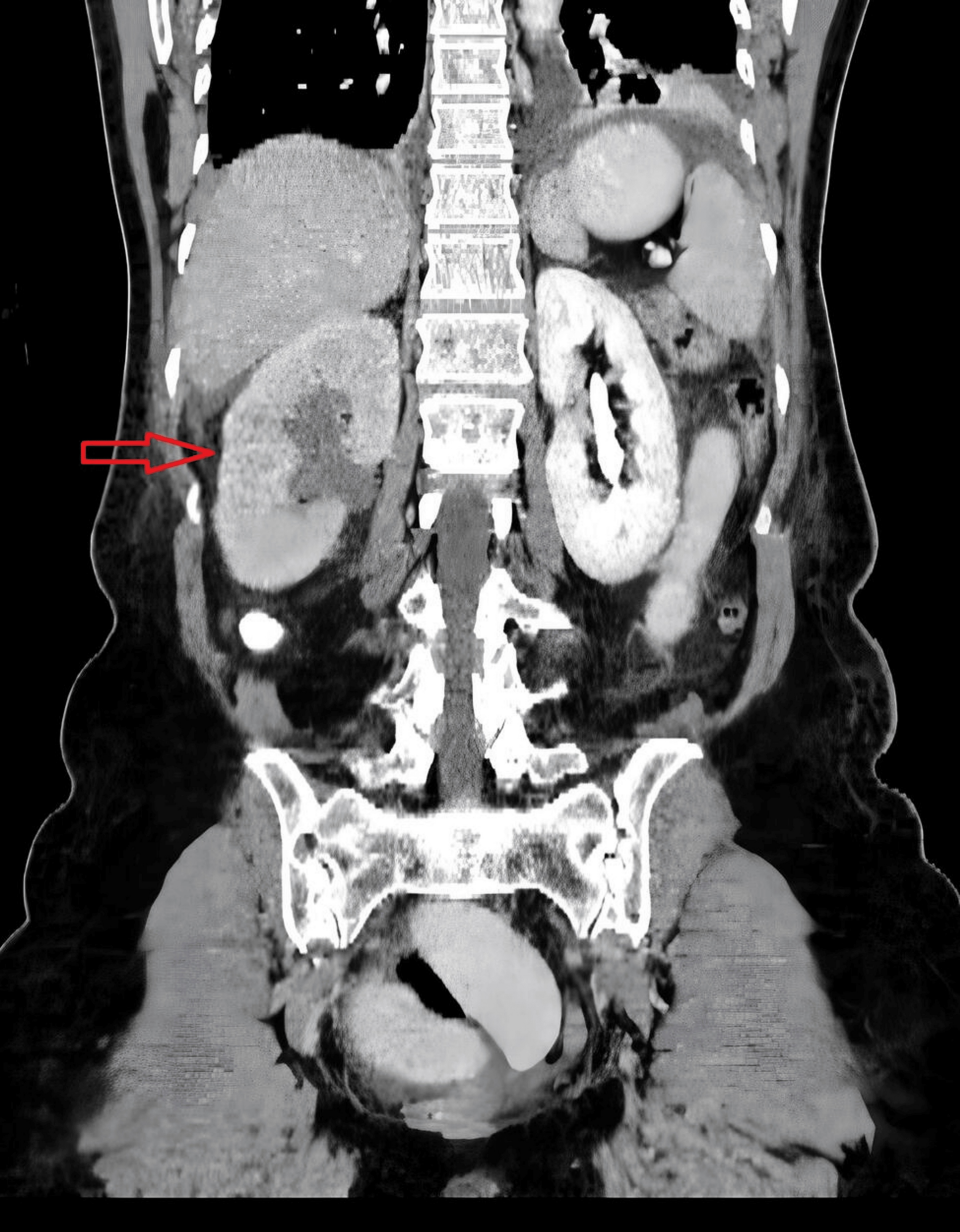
CT showing right-sided hydronephrosis with perinephric stranding and absence of excretion in the right urinary tract (red arrow)
